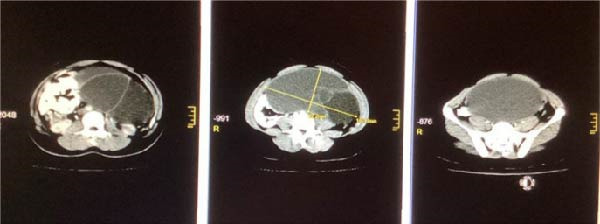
Computed tomography scan with contrast showing a huge cystic mass arising from the left adnexa.
